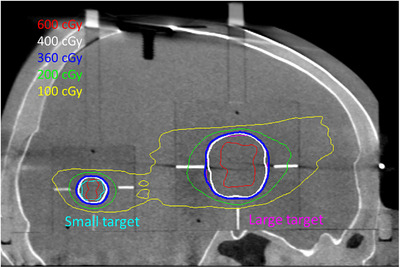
Planned dose distribution on the stereotactic CBCT for G‐Frame E2E testing for the large and small targets
